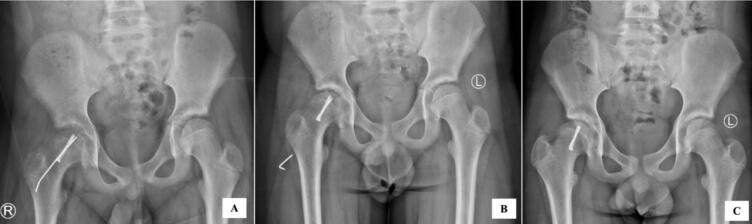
Radiographic images during follow up. (A) in 2 months after surgery, radiograph showed maintenance of the position of the right proximal femoral physis with pain free and full range of motion of the right hip, thus patient was allowed to fully bear weight. (B) at the follow up of 5 months after surgery, radiograph showed broken k-wire, however the reduction was maintained. Patient had been free of pain and had full hip range of motion. (C) at the final follow up of 18 months after surgery, radiograph showed maintenance of the reduction with healing of the right proximal femoral physis and no sign of AVN. Patient had been free of pain and had full hip range of motion, thus patient was allowed to have full activity.
